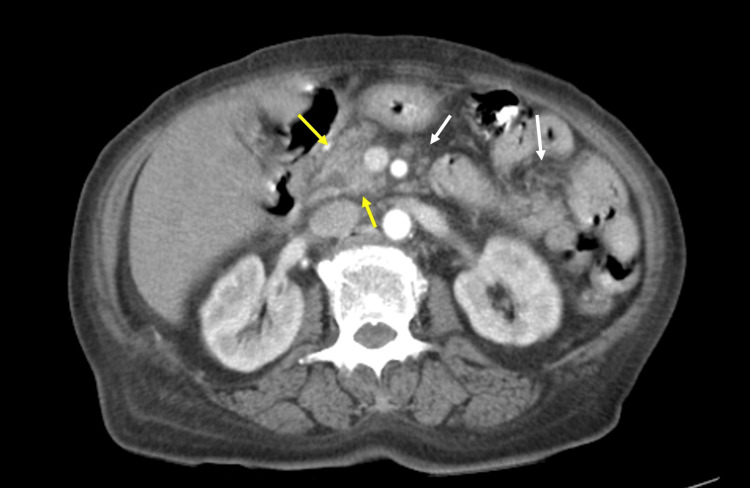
The result of contrast-enhanced abdominal computed tomography (CT). Contrast-enhanced abdominal CT showing enlargement of the pancreatic head (yellow arrows) and increased concentration of the peripancreatic fat tissues (white arrows).
